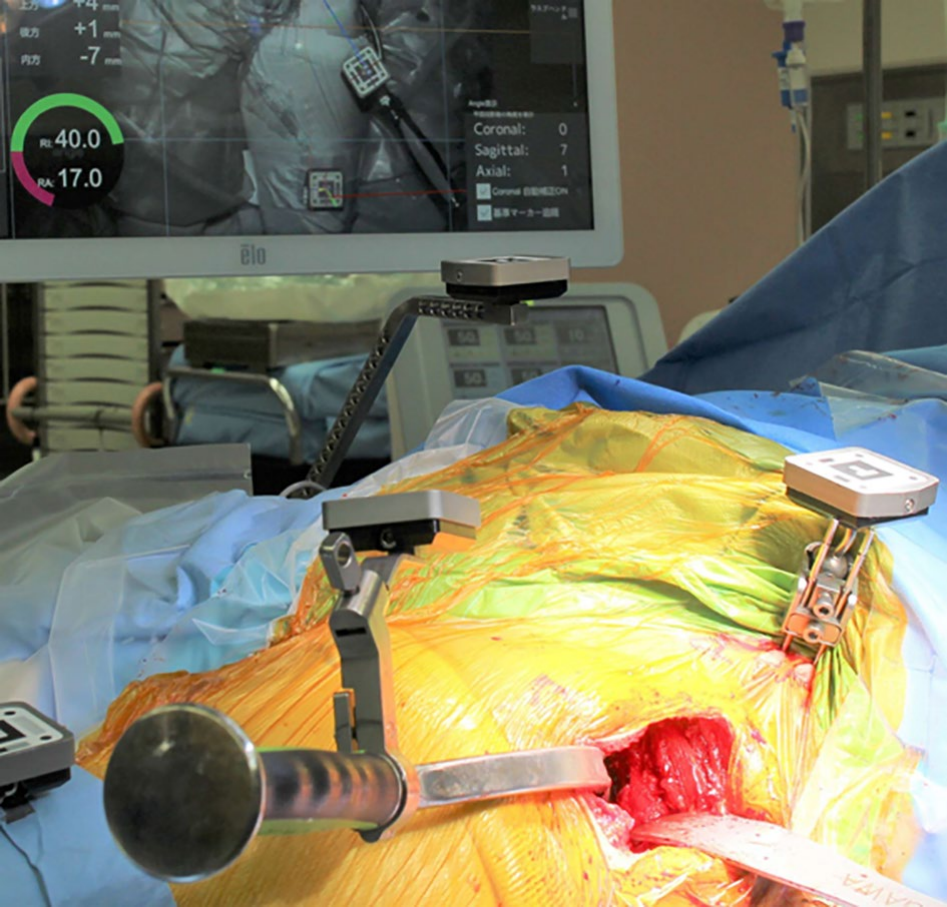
Cup insertion process using the augmented reality (AR) computed tomography (CT)-based navigation system.
